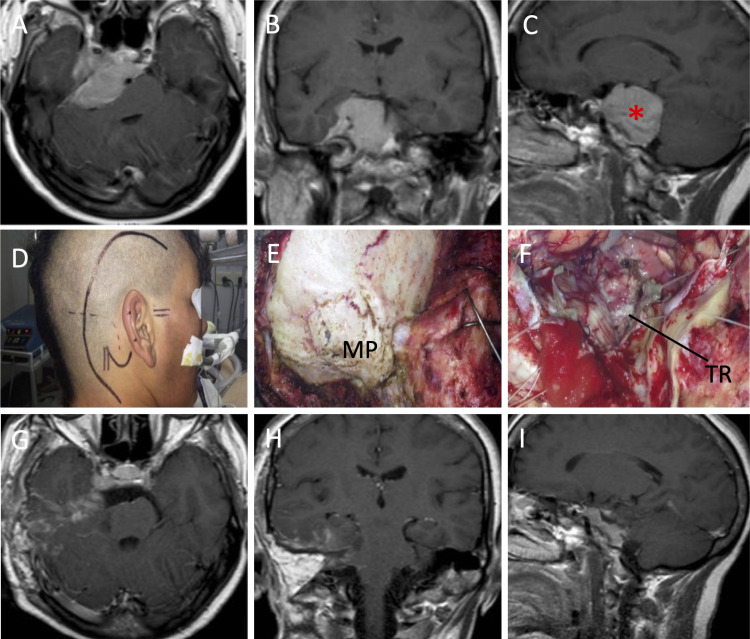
Surgical application of CPSA in a semi-sitting position A-C: Preoperative MRI, D: Incision, E: Bone exposure, F: View after tumor resection, G-I: Postoperative MRI. Asterisk: petroclival meningioma (sagital view). CPSA: combined presigmoid-subtemporal approach; MP: mastoid process; TR: tumor removal
